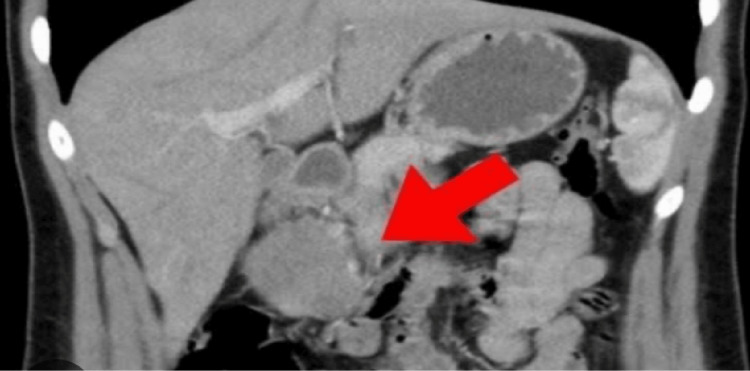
Solid pseudopapillary neoplasm of the pancreatic head on the coronal CT scan
